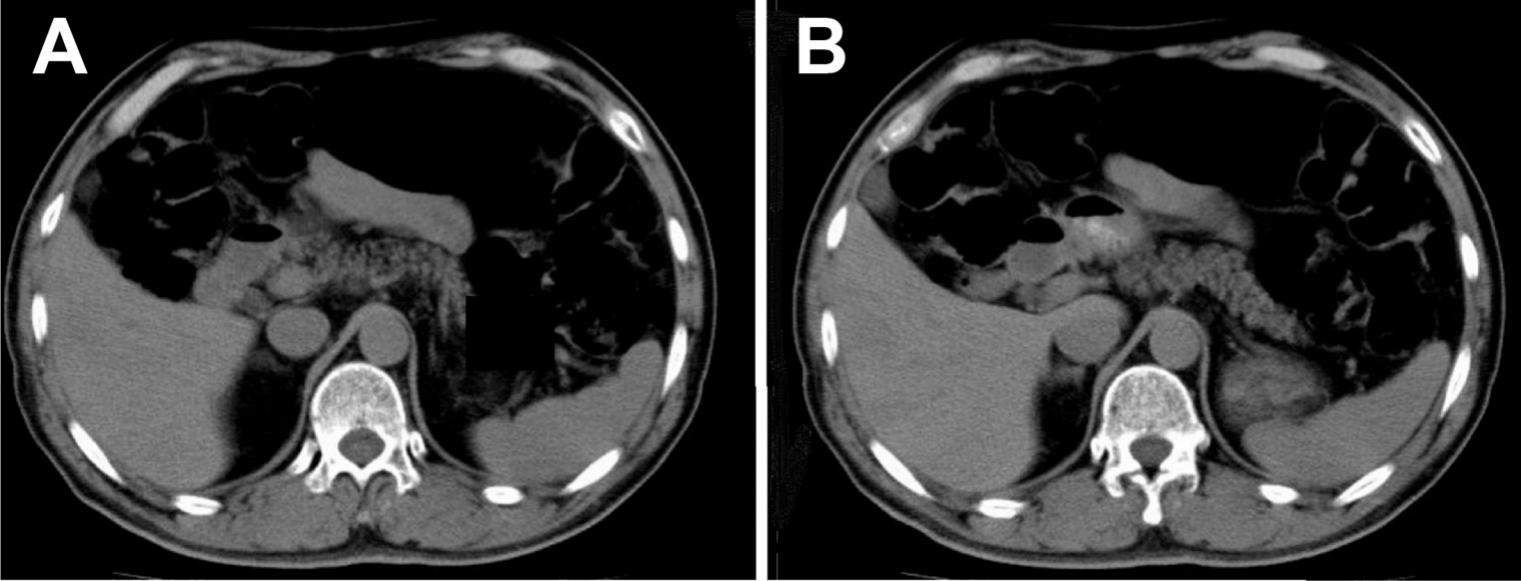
CT Images of the pancreas in a 63-year-old man (A) (B). The patient was relatively lean (body mass index = 22.5 kg/m^2^, visceral fat area = 61.6 cm^2^). The pancreas showed changes in fatty composition (mean CT value of pancreas = 24.4 Hounsfield units, mean CT value of pancreas / CT value of spleen = 0.53). The participant had pancreatic endocrine impairment (increment of C-peptide immunoreactivity level at 6 min after an intravenous injection of 1 mg glucagon = 1.39 mmol/L) and pancreatic exocrine impairment (urinary p-amino benzoic acid excretion rate = 62.5%).
